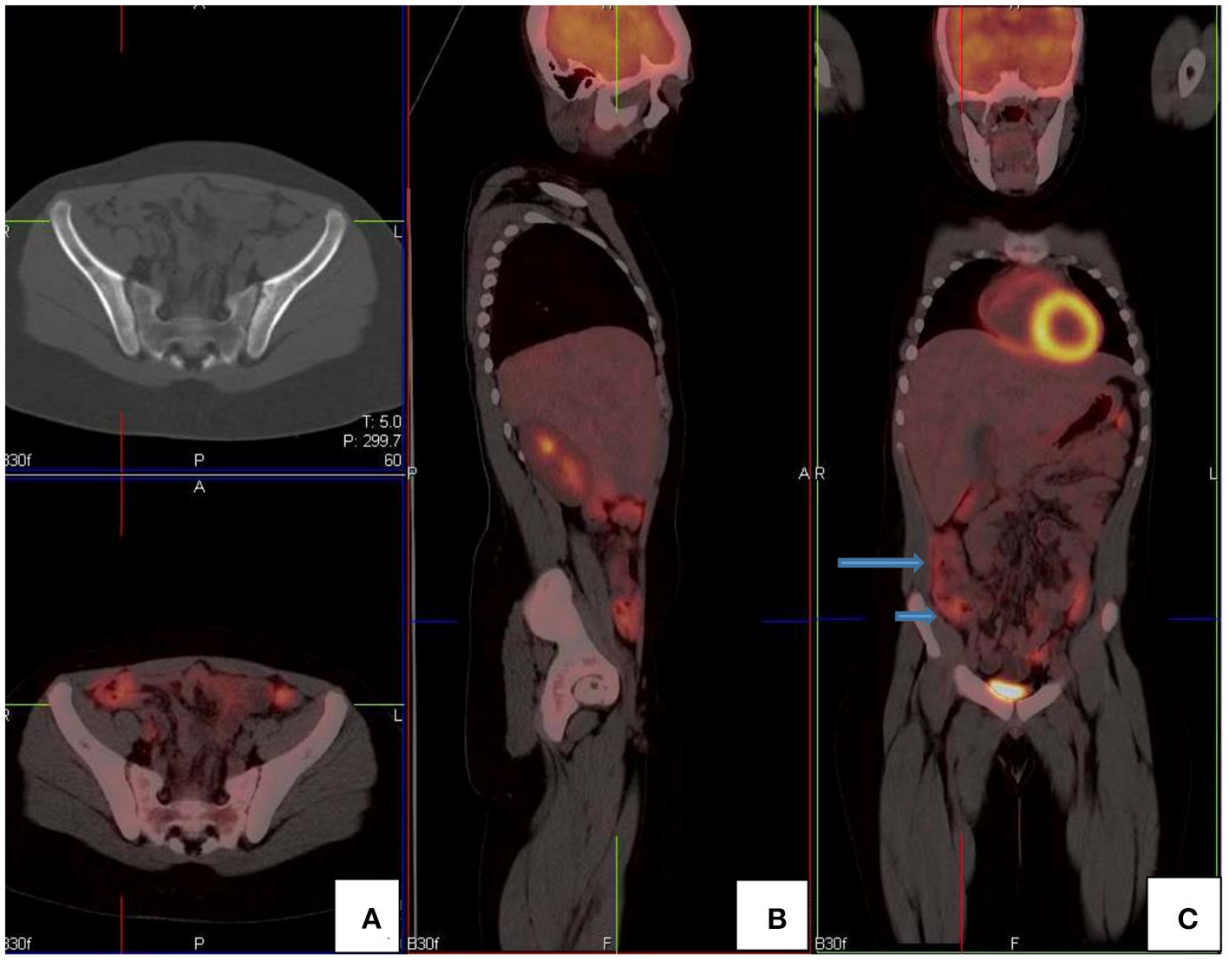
FDG PET/CT (A) axial, (B) sagittal and (C) coronal images show markedly increased FDG uptake in the normal right colon (long arrow). Note the more intense uptake in the cecum due to the higher amount of lymphoid tissue (short arrow).
